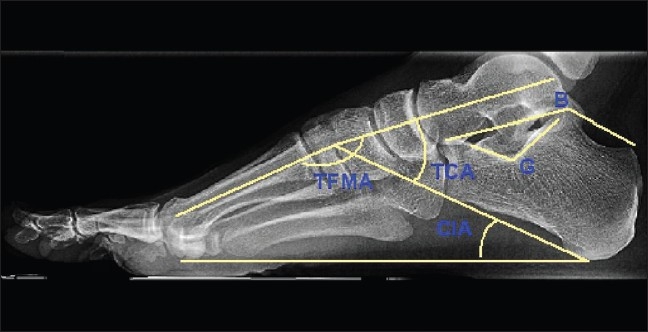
X-ray foot and ankle (lateral view) showing measured and assessed angles. B - Böhler's angle (20-40°), G - Gissane's angle (120-145°), TCA – talocalcaneal angle (25-50°), TFMA – talus first metatarsal angle (0 – 10°), CIA – calcaneal inclination angle (20 – 25°)
